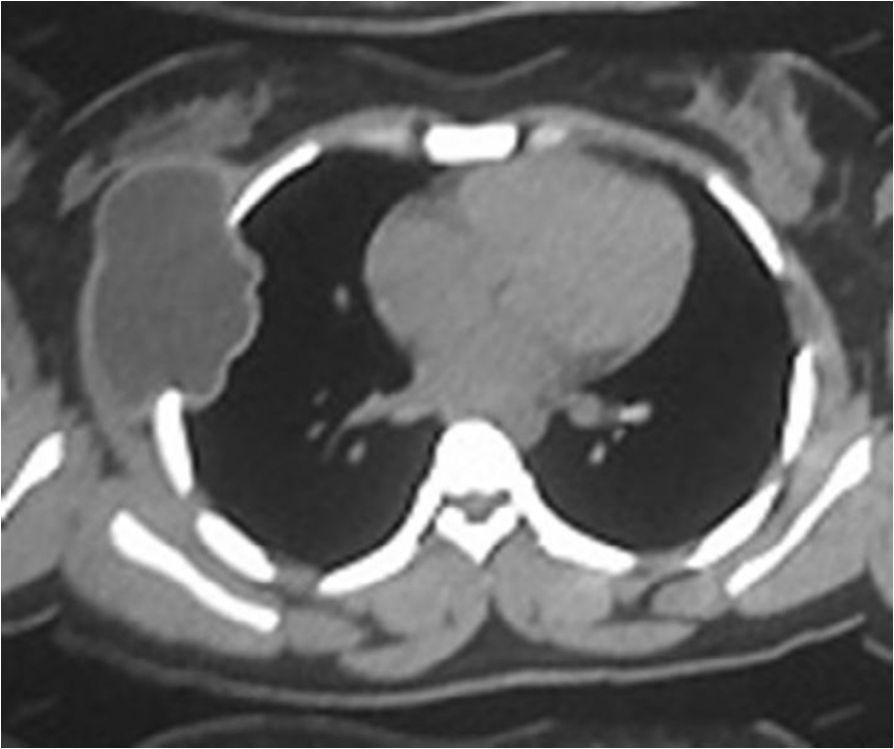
CT scan of a patient with chest wall tumor.
